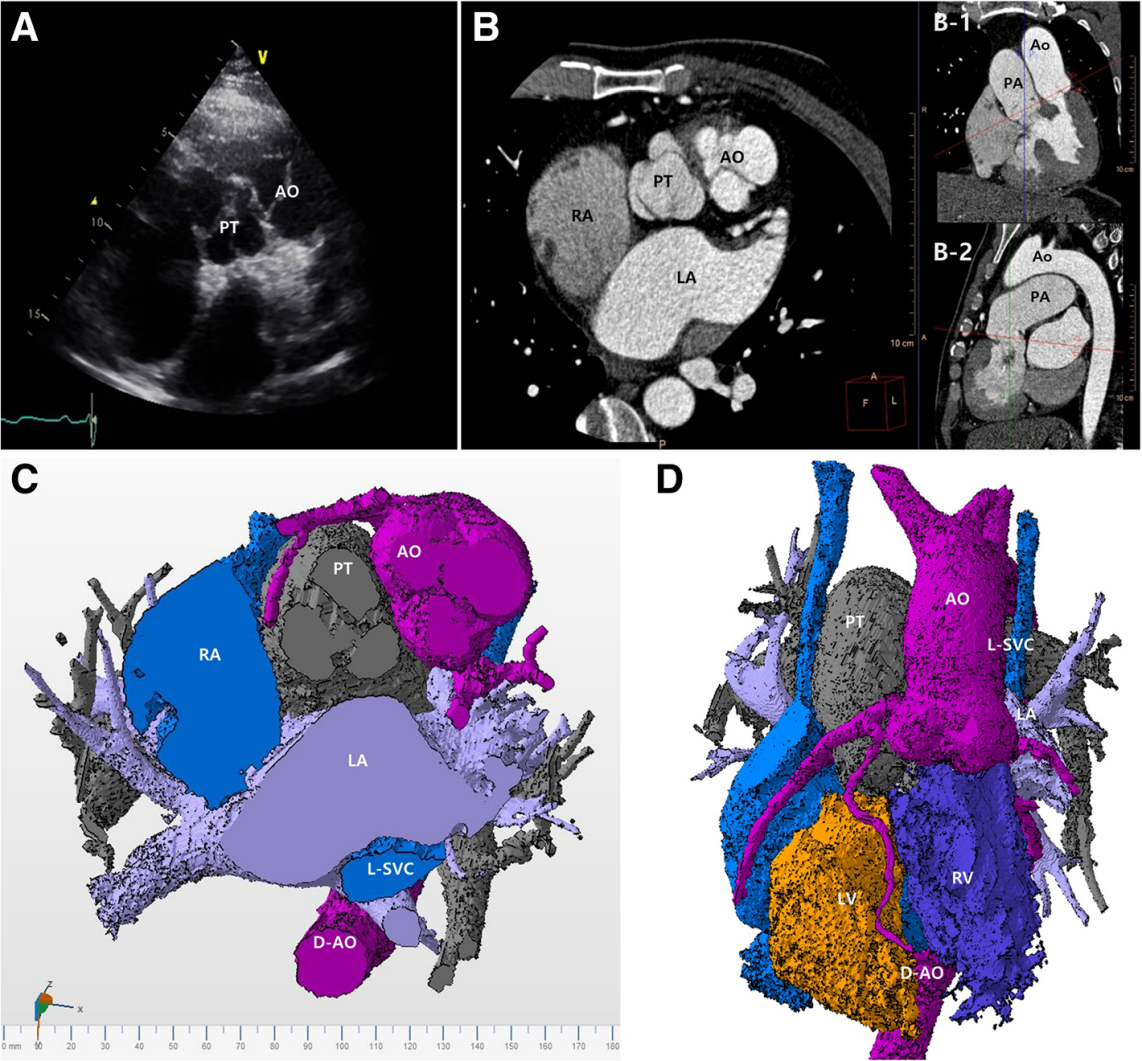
a Transthoracic echocardiography shows the pulmonary trunk in the center position of the parasternal short axis view, which was opened to the right-sided ventricle, and the aortic valve in the 1 o’clock position, suggesting the transposition of the aorta and pulmonary trunk. b Cardiac computed tomography images at the cutting planes shown in *red lines* in B-1 and B-2 demonstrate the aorta and pulmonary trunk to be in similar positions to those shown by transthoracic echocardiography. c The cranial part of the three-dimensional model of the cardiac computed tomography at a similar plane as that in (a and b). d The parallel arrangement of the aorta and pulmonary trunk are shown in a three-dimensional model. *AO* aorta, *D-AO* descending aorta, *LA* left atrium, *L-SVC* left superior vena cava, *LV* left ventricle, *PA* pulmonary artery, *PT* pulmonary trunk, *RA* right atrium, *RV* right ventricle
